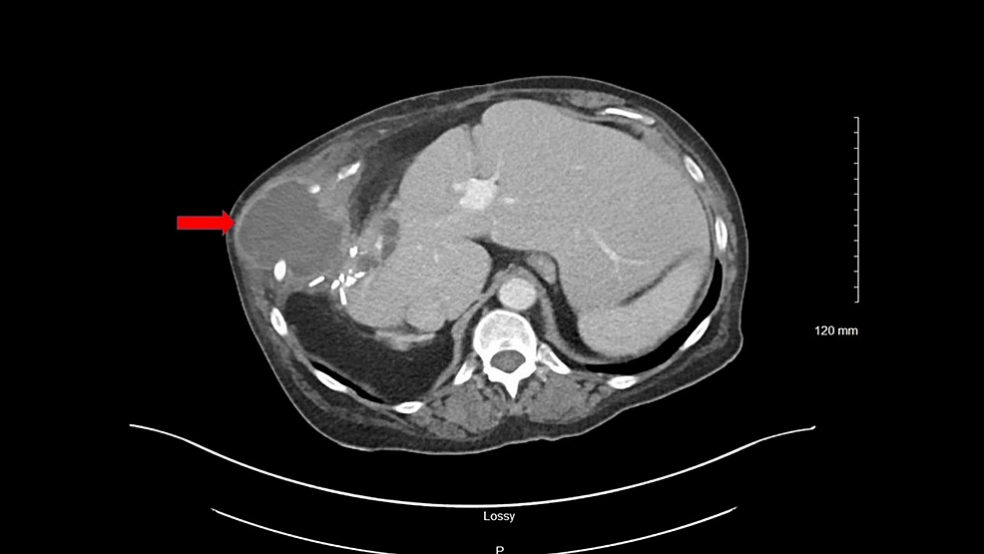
CT chest showing right chest wall abscess CT - Computed tomography
